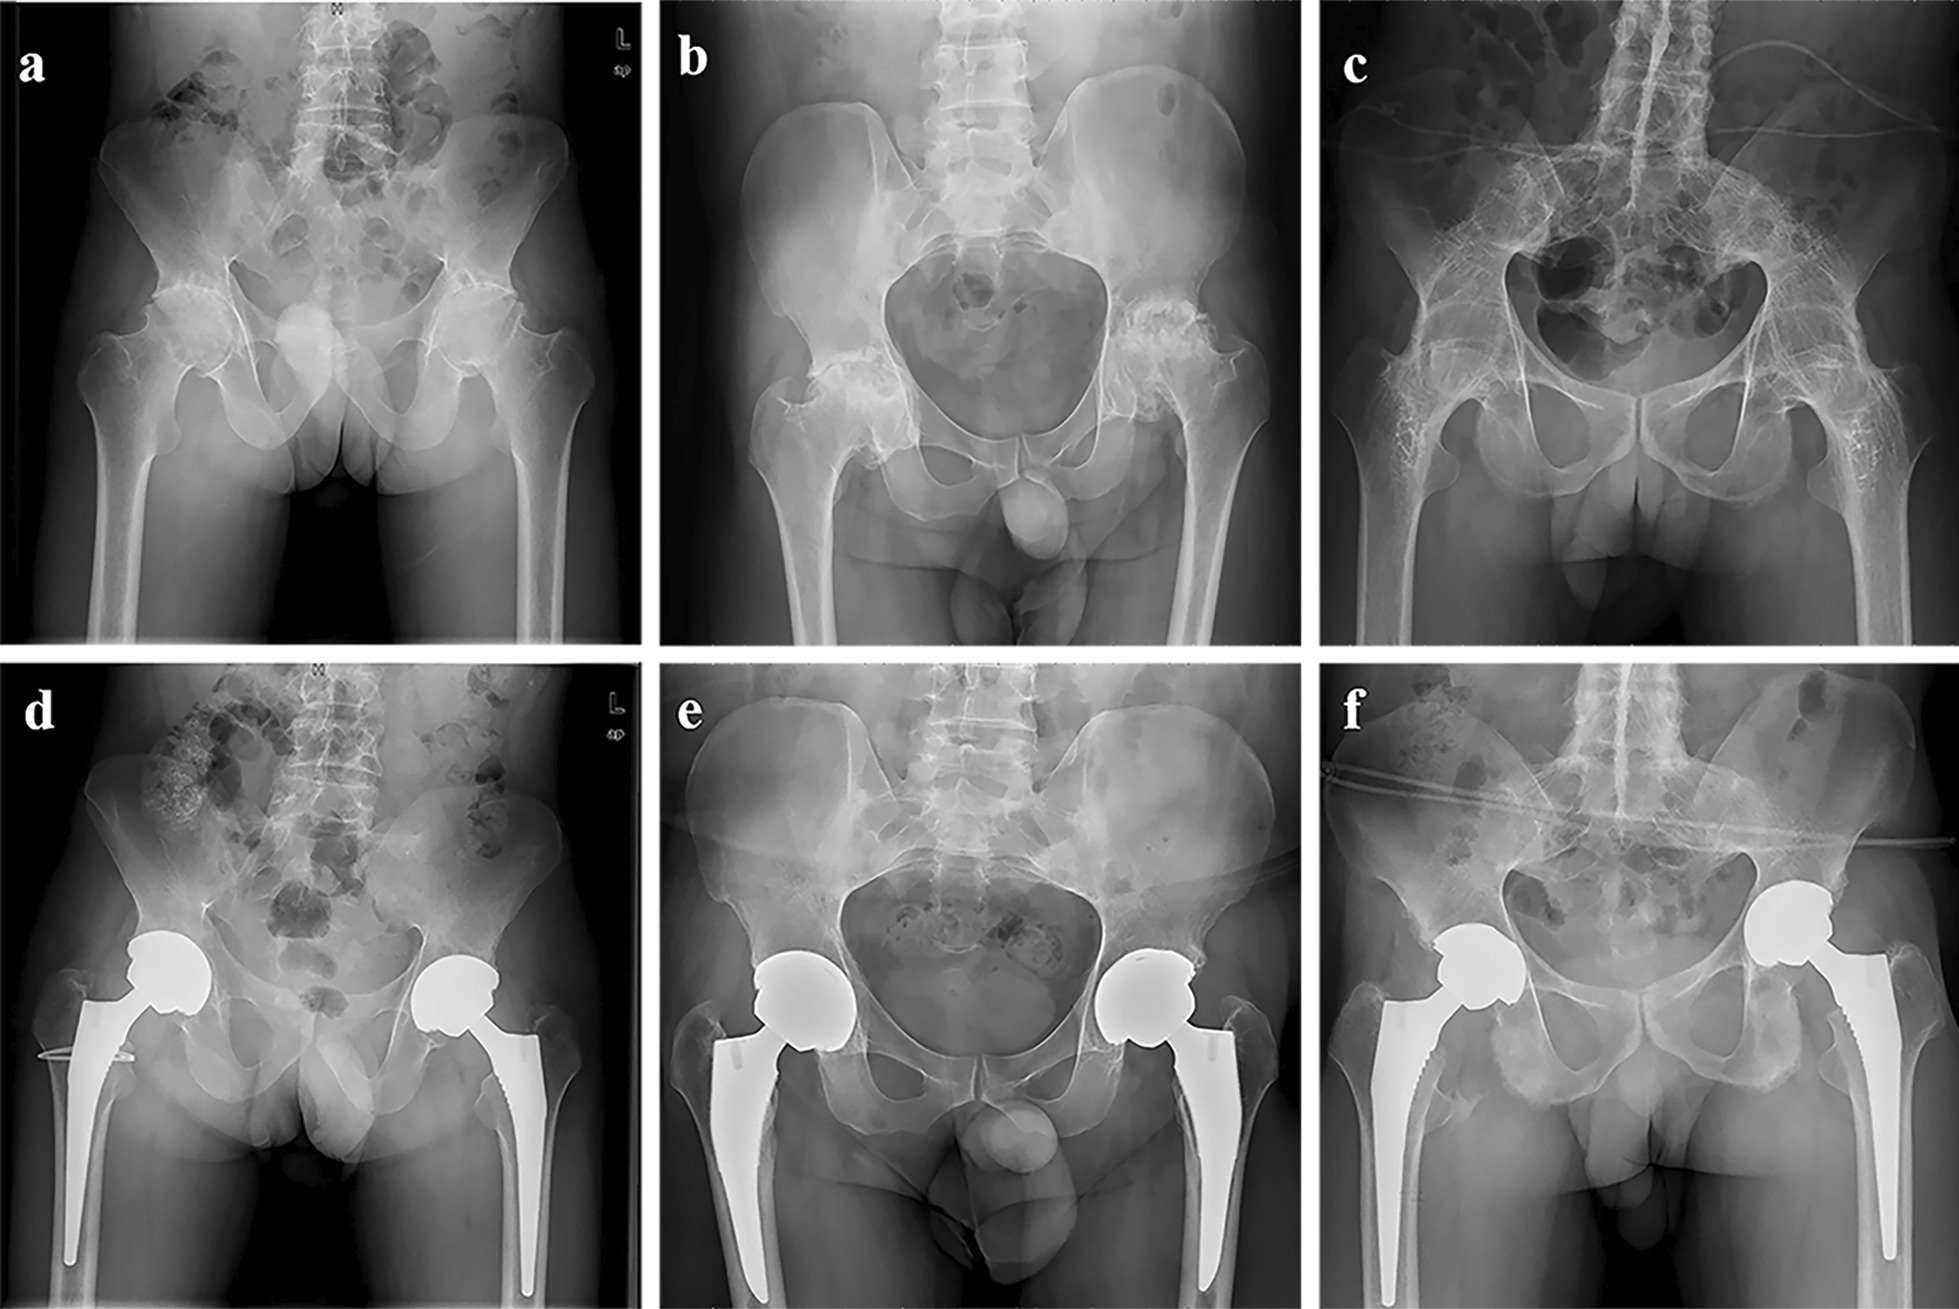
Case presentation of bilateral cementless bilateral total hip arthroplasty for three different degrees of hips involvement with ankylosing spondylitis. a The radiograph showed bilateral hips involved preoperatively and physical examination showed no total loss of range of motion. b The radiograph showed bilateral hips involved and no trabecula bridged the hip joint plane preoperatively and physical examination showed total loss of range of motion. c The radiograph showed bilateral hips involved and trabecula bridged the hip joint plane preoperatively and physical examination showed total loss of range of motion. d The pelvis radiograph at the latest follow-up (78 months for both hips) for Fig. 1a showed excellent prosthesis position, no loosening and migration of the component. e The pelvis radiograph at the latest follow-up (82 months for right hip and 81 months for left hip) for Fig. 1b showed excellent prosthesis position, no loosening and migration of the component. f The pelvis radiograph at the latest follow-up (80 months for both hips) for Fig. 1c showed excellent prosthesis position, no loosening and migration of the component
